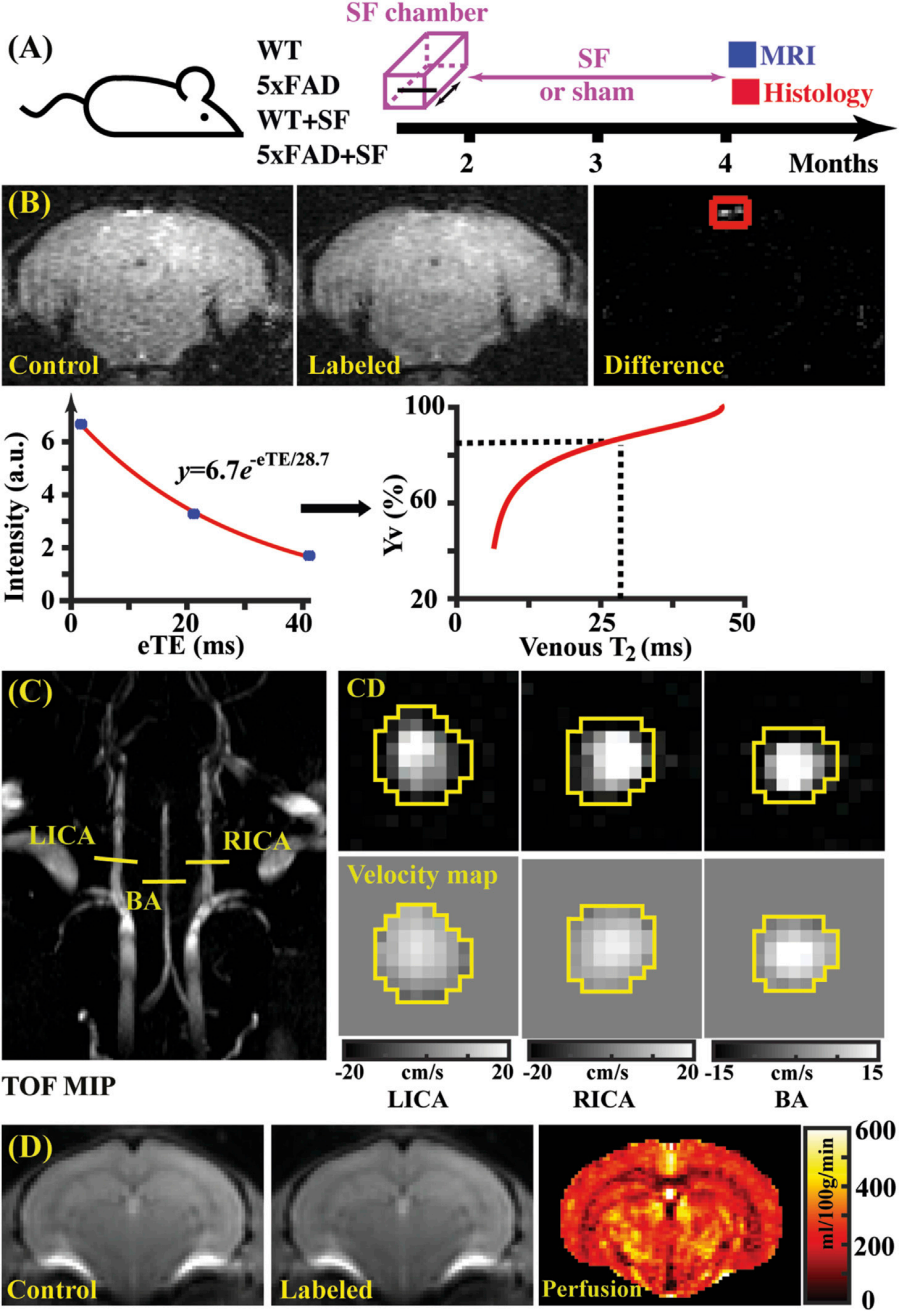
Study design (A) and representative images of TRUST (B), PC (C), and pCASL (D) MRI. (B) Top panel: control, labeled, and difference images for TRUST obtained at the eTE of 0.25 ms. Sinus confluence was marked by a red square. Bottom panel: signal intensities of venous blood as a function of eTE. The fitted venous T_2_ can be converted into oxygenation by reference to the T_2_-Yv calibration plot (Li and van Zijl, 2020). (C) Left panel: maximal intensity project (MIP) of time-of-flight (TOF) MRI to visualize the feeding arteries. Right panel: complex difference (CD) images and velocity maps for the three feeding arteries (LICA, RICA, and BA). (D) Control, labeled, and perfusion images from the pCASL MRI.
